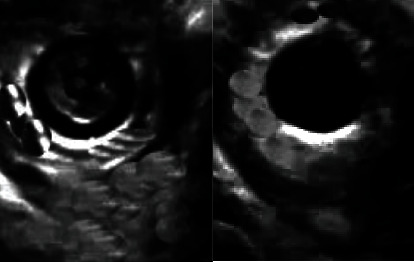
The 3D ultrasound image of internal and external AS of a primipara (aged 26 years old).
